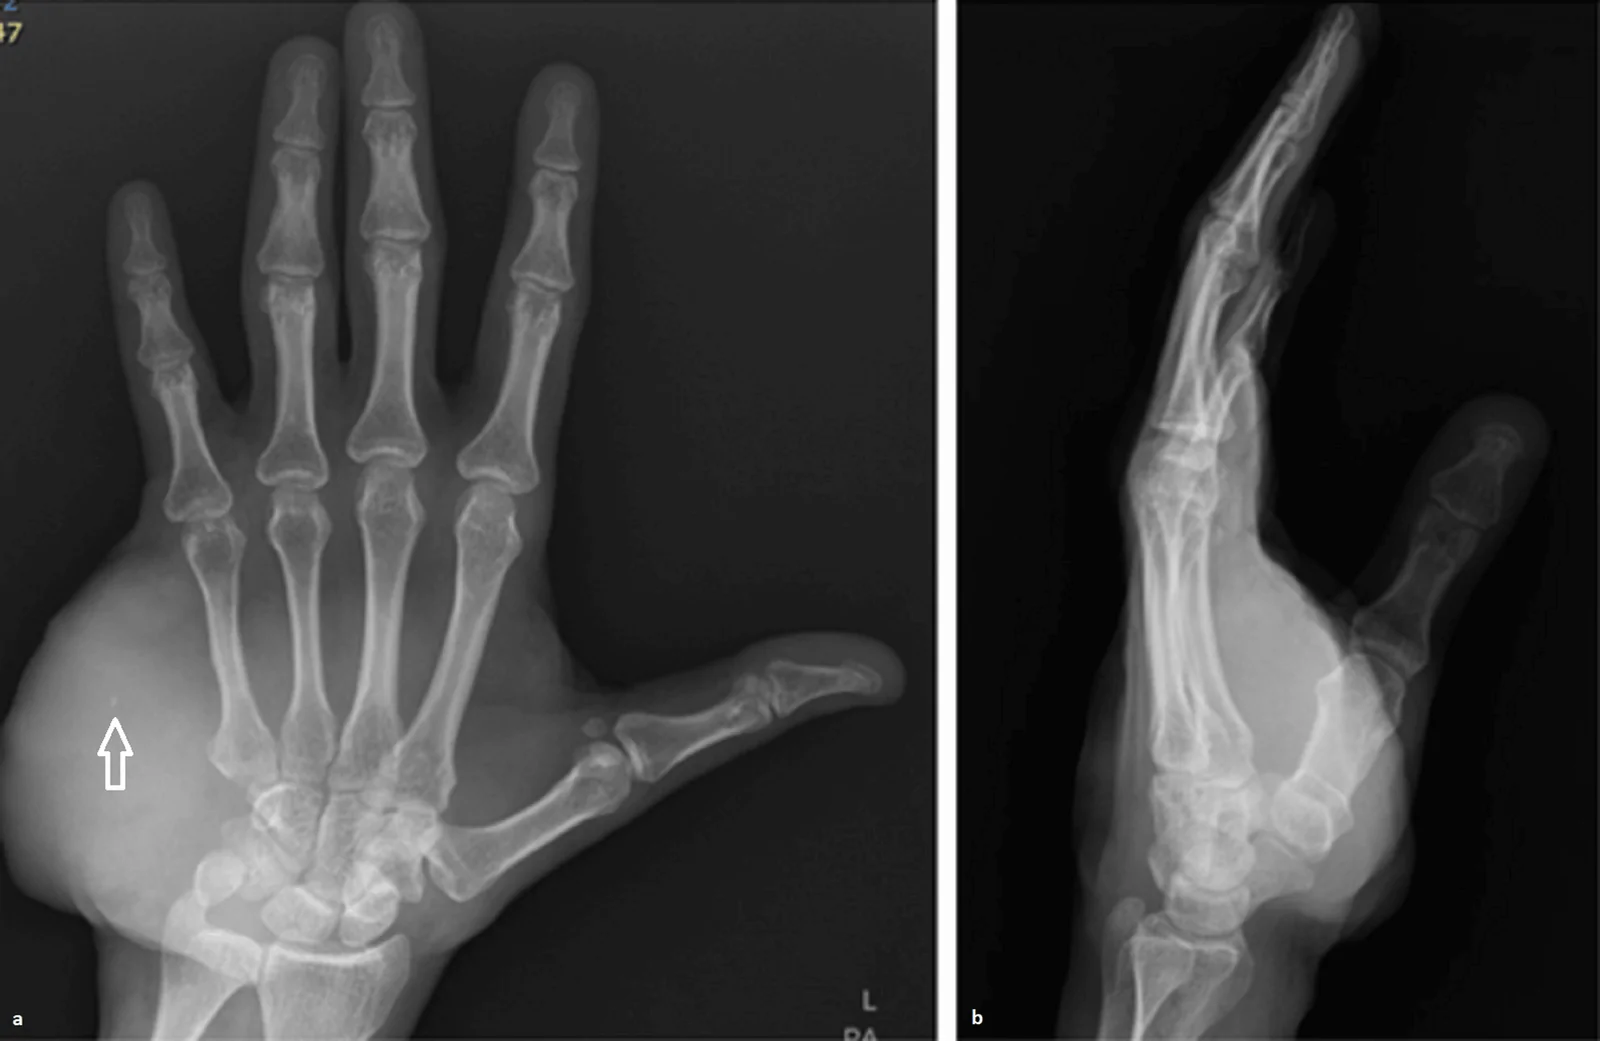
X-ray left hand PA (A) and lateral (B) Lobulated homogeneously dense soft tissue lesion seen along the ulnar aspect of the hand. Small round calcification is noted within the lesion (arrow).
